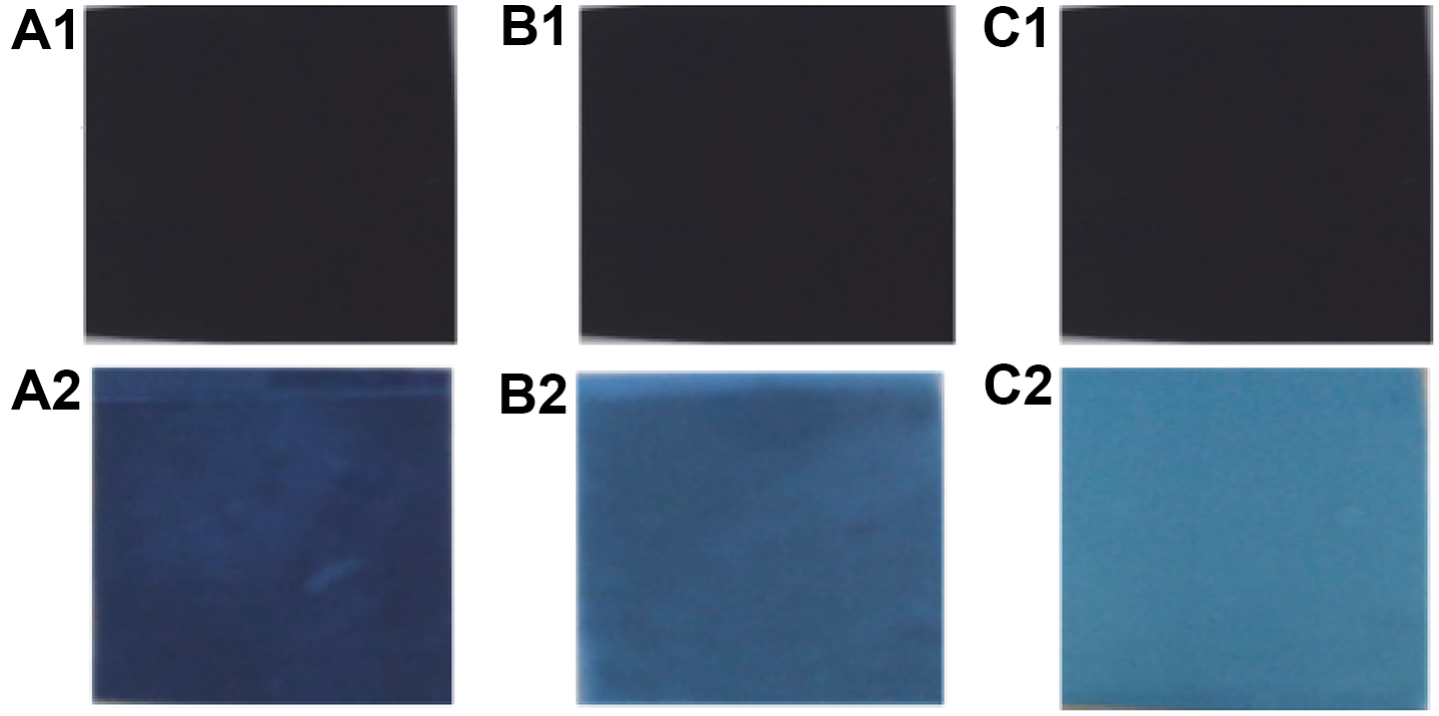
X-ray photographic film gelatinolysis for silver recovery using SpSKF4 protease from *G. thermoglucosidasius* SKF4: Letter A1, B1 and C1 shows X-ray film before exposure to enzyme treatment, A2 after exposure to partially purified *B. licheniformis* 2D55 protease for 3 h (positive control), B2 after treatment with Glycine-NaOH buffer only (Negative control), and C2 after exposure to SpSKF4 protease enzyme for 3 h. The experiment were performed in triplicates. There was no observable differences in the replicate for each experiment.
